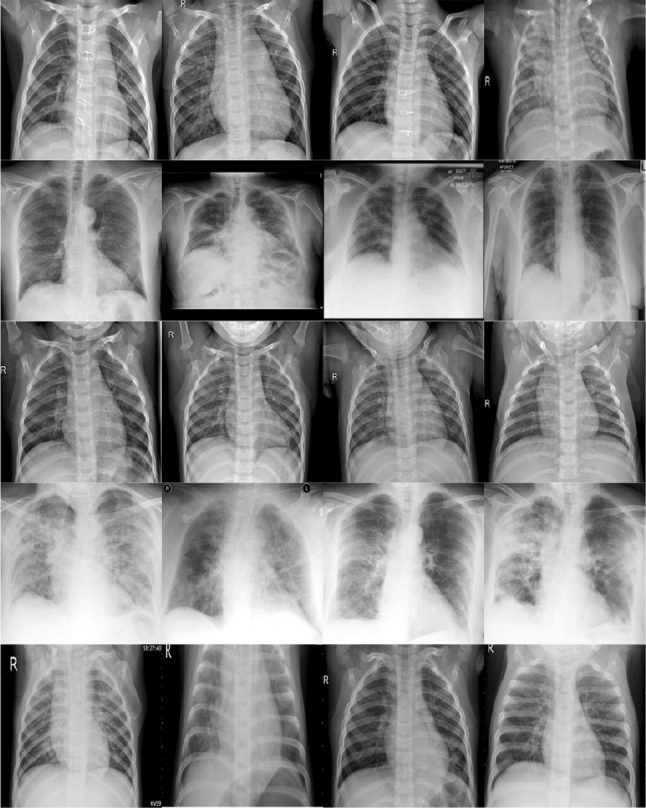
Some samples from our dataset which includes an image from each class. Each row represents x-ray images from each class. From top row to bottom: bacterial pneumonia, COVID-19, normal, SARS and viral pneumonia
